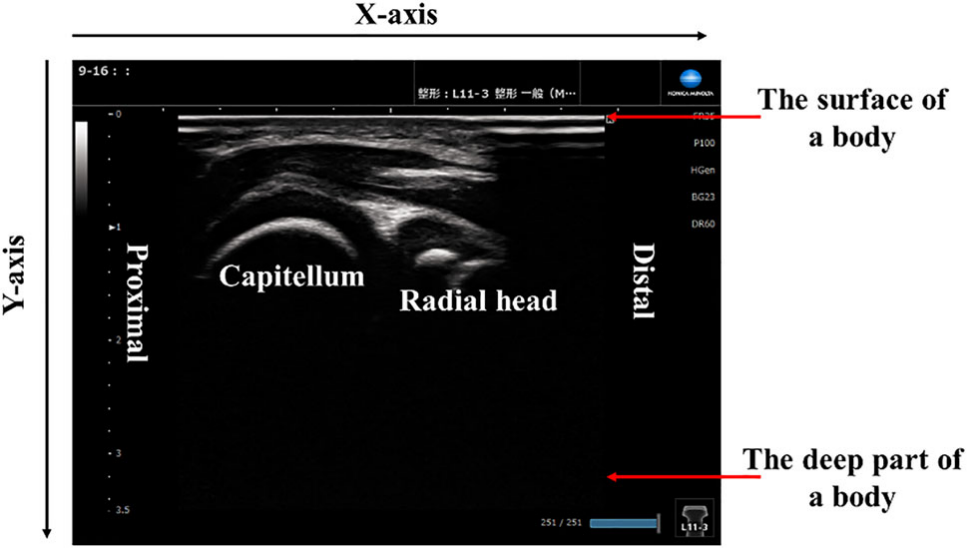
Ultrasound images of the elbow in lateral, posterior, and long-axis imaging directions
